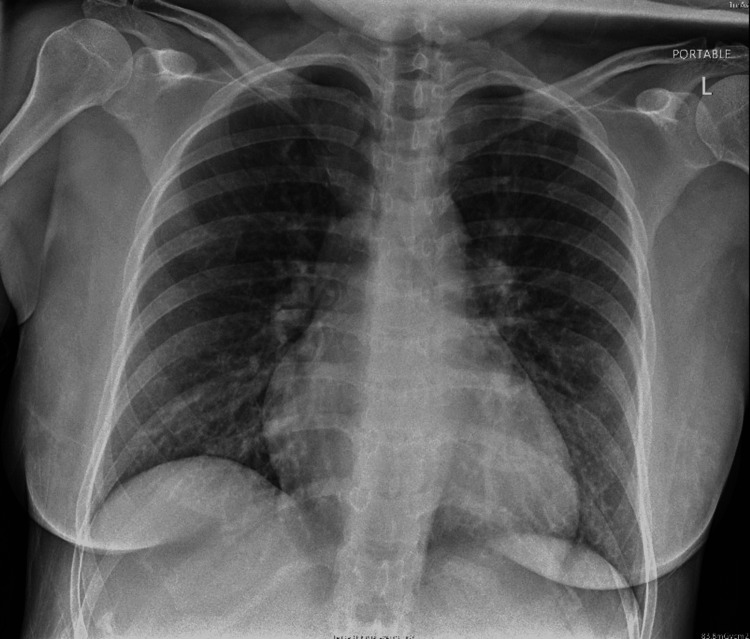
Chest X-ray (AP view) Chest X-ray showing prominent bilateral hilar broncho-vascular marking AP: anterior posterior
